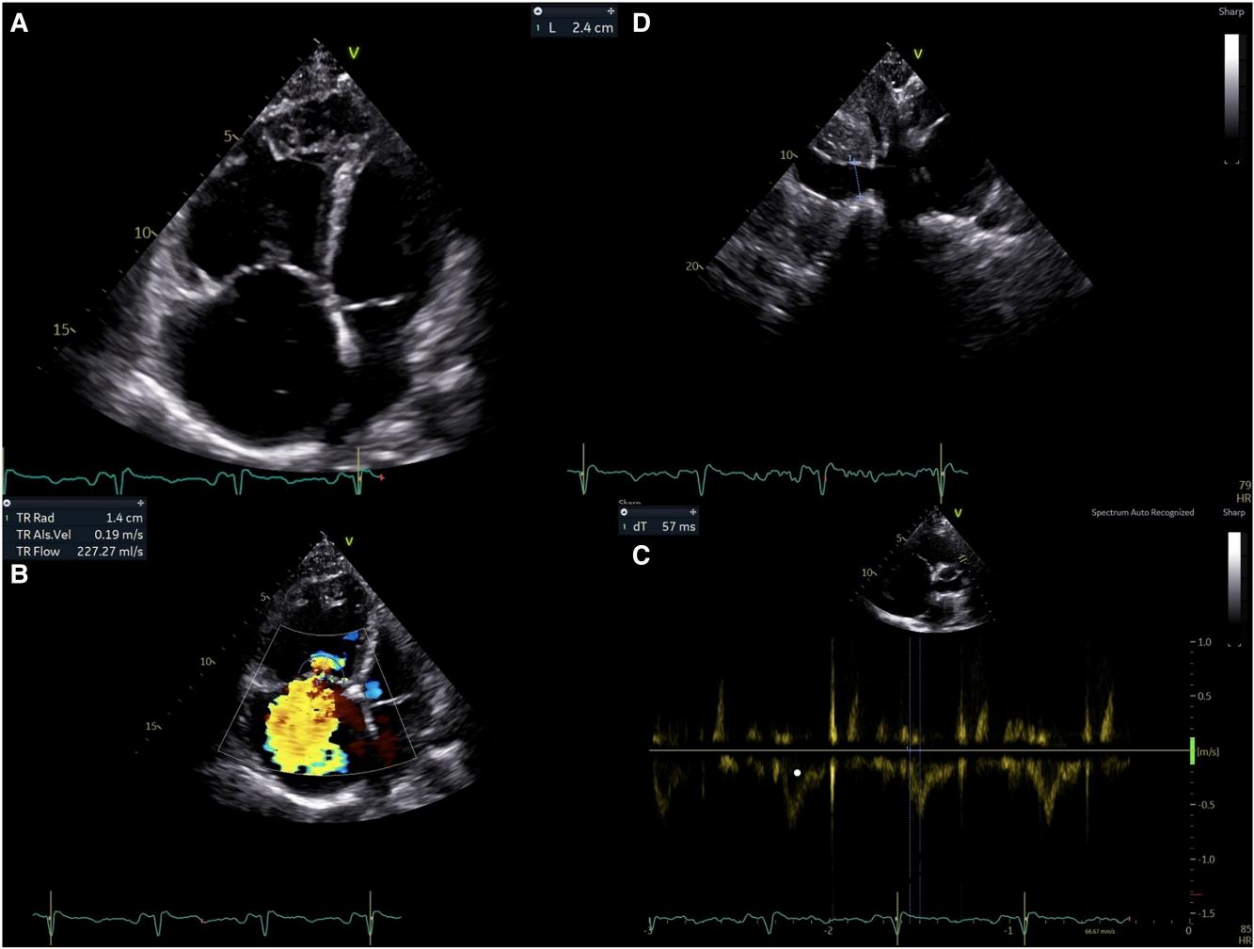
(*A*) Marked right-ventricular dilatation with right-atrial enlargement and interventricular septal flattening, consistent with severe RV pressure overload. (*B*) Severe tricuspid regurgitation with a broad, dense jet filling the right atrium, sPAP estimate of ∼100 mmHg (*C*). Dilated inferior vena cava (≈24 mm) with minimal/absent inspiratory collapse, indicating elevated right-atrial pressure. (*D*) Pulmonary flow (RVOT) pulsed-wave Doppler. Reduced acceleration time (AT ∼57 ms) in the right-ventricular outflow tract supporting severe pulmonary hypertension.
